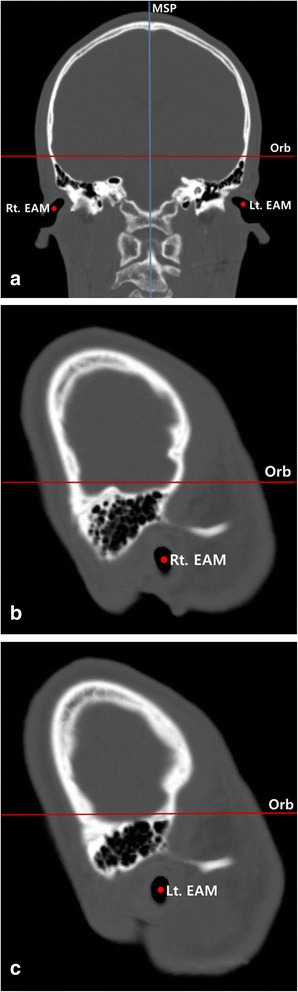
Determination of EAM point on 3D CT image. EAM was defined as a center point of the most outer ovoid soft tissue shadow of the external auditory meatus. a Frontal view. b Lateral of right EAM. c Lateral view of left EAM. *MSP* midsagittal plane, *Orb* orbital plane, *EAM* external auditory meatusMx: the mesiobuccal cusp tip of maxillary first molar, both sides
